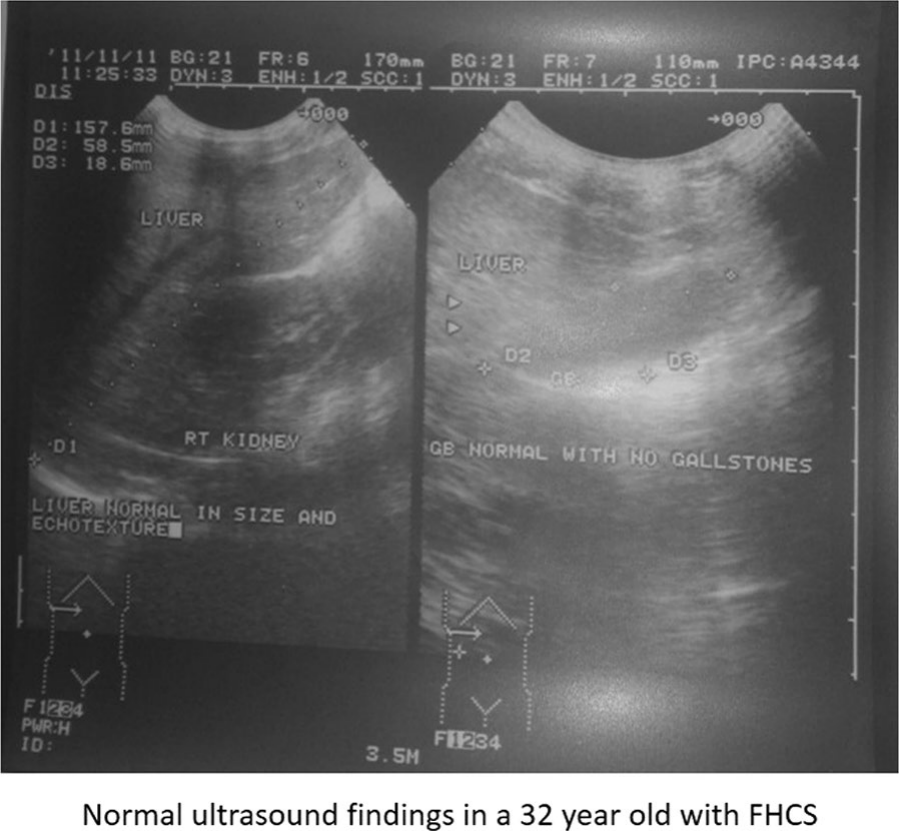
Normal ultrasound findings in a 32 year-old with Fitz-Hugh–Curtis syndrome
